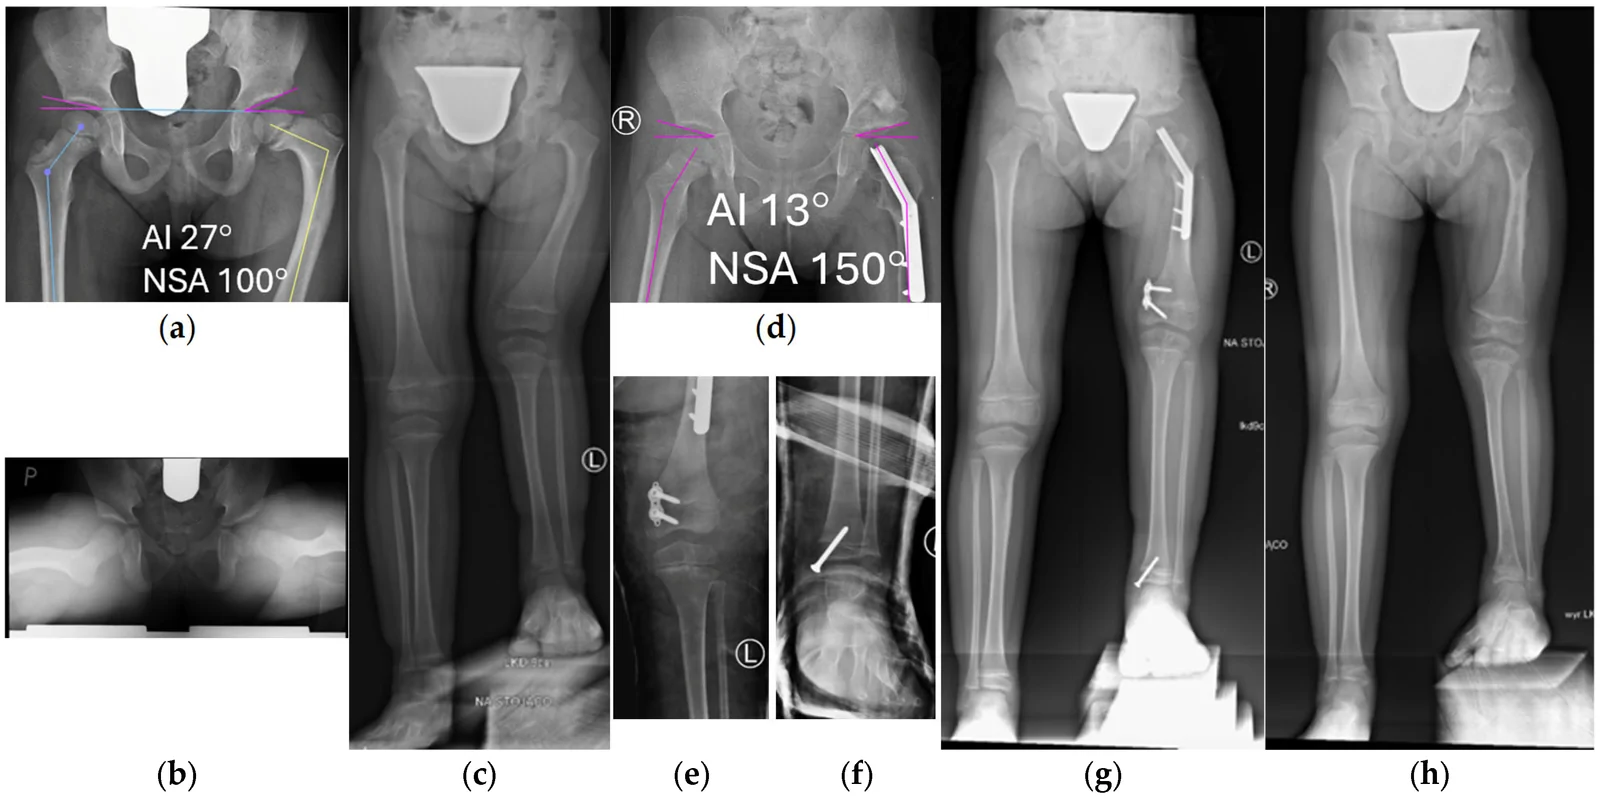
Radiographs demonstrating treatment of left CFD type 1A3: (a) anteroposterior view; (b) reduced femoral anteversion angle; (c) standing radiograph showing knee valgus, proximal migration of the lateral malleolus and 9 cm LLD; (d) pelvic radiograph after SUPERhip procedure; (e) 8-plate fixation for correction of knee valgus; (f) medial malleolar hemiepiphysiodesis performed to prevent ankle valgus; (g) standing radiograph obtained 1 year later showing restoration of the femoral axis and reconstructed hip prior to hardware removal; (h) standing radiograph after hardware removal. LKD—left lower limb, NA STOJĄCO—standing position, L—left, R—right, AI—acetabular index, NSA—neck-shaft angle.
